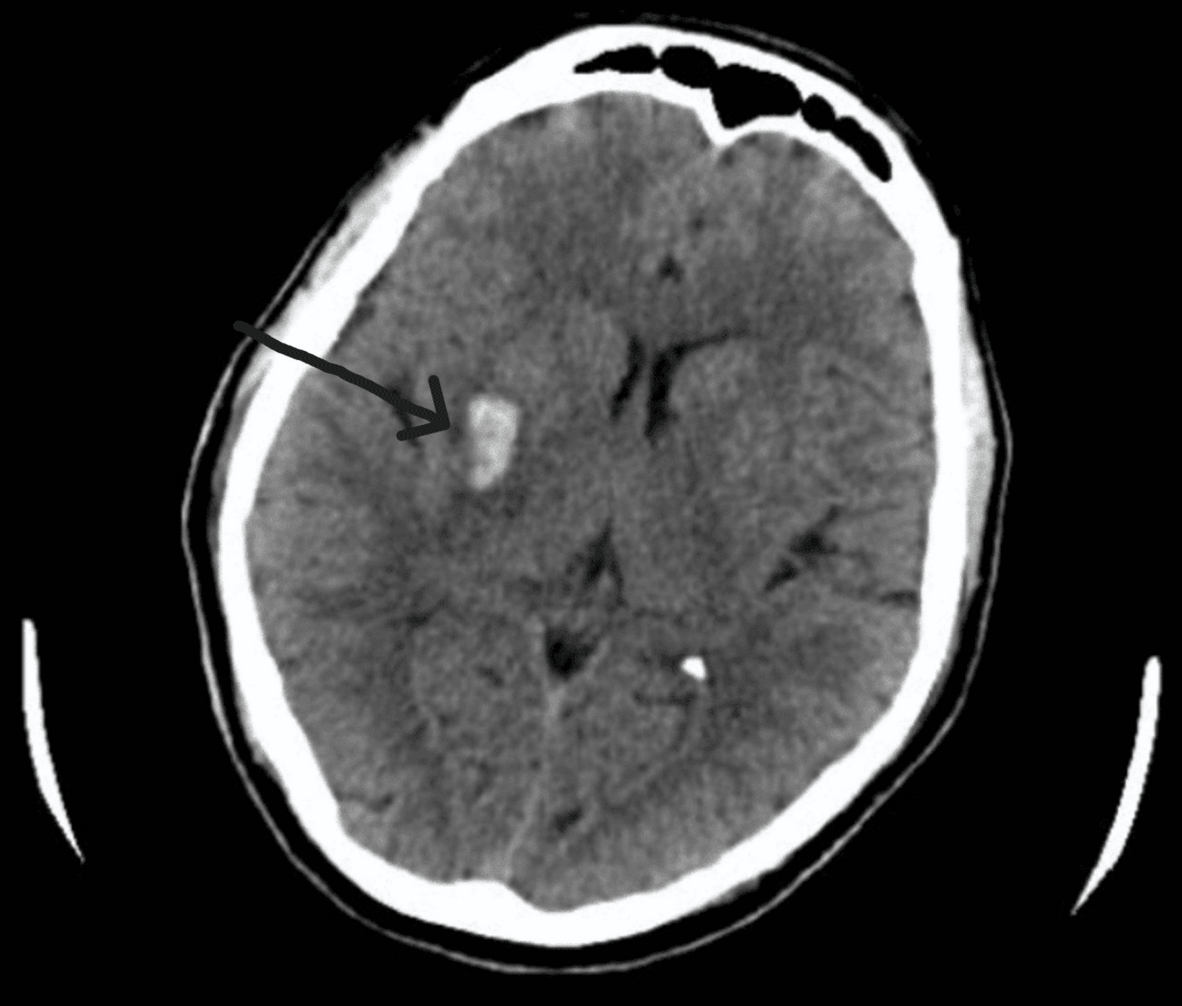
Non-contrast CT of the brain showing a right-sided intracerebral hemorrhage in the basal ganglia region, with minimal surrounding mass effect. CT: computed tomography
